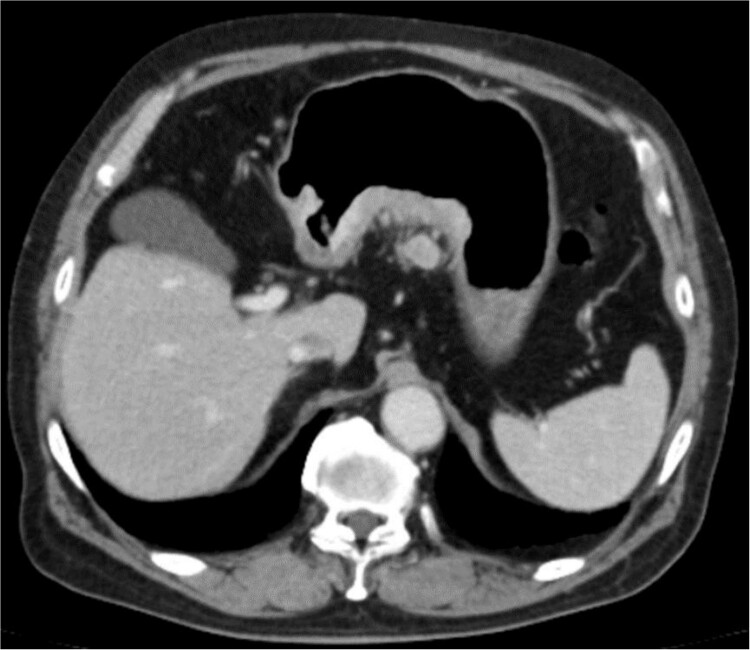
Clinical findings of contrast-enhanced CT. CT revealed the thickness of gastric wall and enlarged lymph nodes of lesser curvature side.
